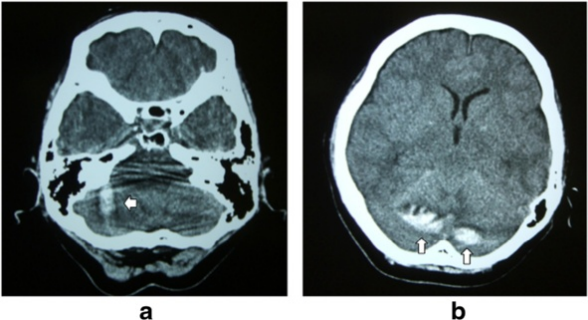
Non-enhanced plain computed tomography scan of the head taken 13 hours after spinal surgery, demonstrating an acute cerebellar hemorrhage in the superior folia of the cerebellar hemispheres (white arrows). (a) At the low cerebellum level, (b) At the cerebral peduncles level.
